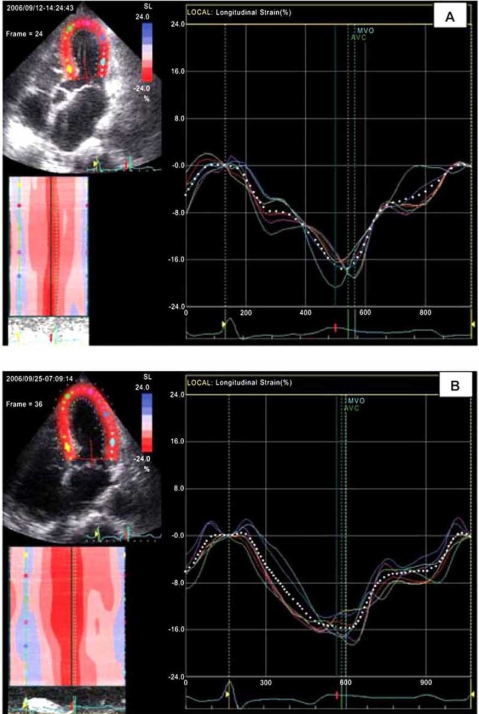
Longitudinal global strain (dotted white curve) and regional longitudinal strain curves (distinctively colored curves for 6 left ventricular wall  segments) obtained from apical 4-chamber views by speckle-tracking 2D-strain imaging in a patient with Takotsubo cardiomyopathy. Although during catecholamine induced severe LV dysfunction with apical ballooning (A) the apex appeared nearly akinetic (no visible relevant inward movement) the longitudinal strain curves showed the same uniform longitudinal shortening as after recovery (B), when also visually no regional wall motions were detectable. Thus, the visual analysis of inward movement used in conventional echocardiographic examinations can be misleading in the evaluation of regional myocardial contraction because it can not exclude the existence of longitudinal shortening (not visible with the naked eye) in the apparently akinetic region [cf. Dandel *et al.* International Journal of Cardiology 2008].
